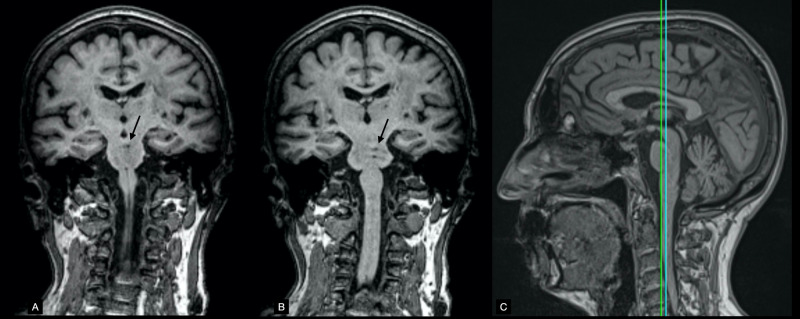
Brainstem MRI Panels A and B show T1-weighted images of two coronal planes. Notice the hypointense lesions seen left of the midline on both images (black arrows). Panel C shows the positions of A (anterior, green line) and B (posterior, blue line) on a T1-weighted image of the midline sagittal plane.
